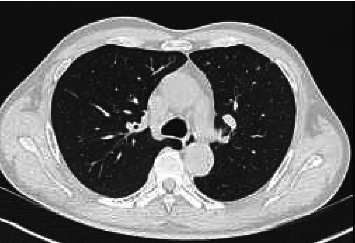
Two months later, chest CT scan showing complete resolution of infiltrate from posterior pneumonia of the upper left lobe.
